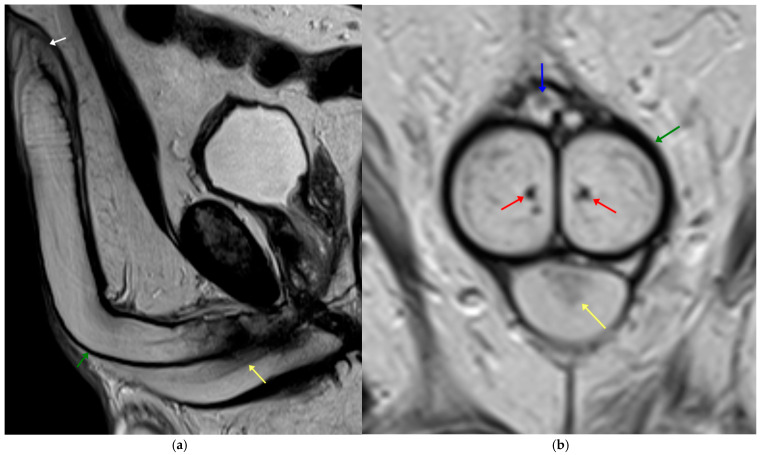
Normal penile anatomy on T2-weighted MRI. (a) Sagittal and (b) coronal images. Green arrows indicate the tunica albuginea; yellow arrows denote the urethra centrally located within the corpus spongiosum. The glans penis is marked with a white arrow. Red arrows identify the cavernosal arteries, and the blue arrow highlights the superficial dorsal vein.
